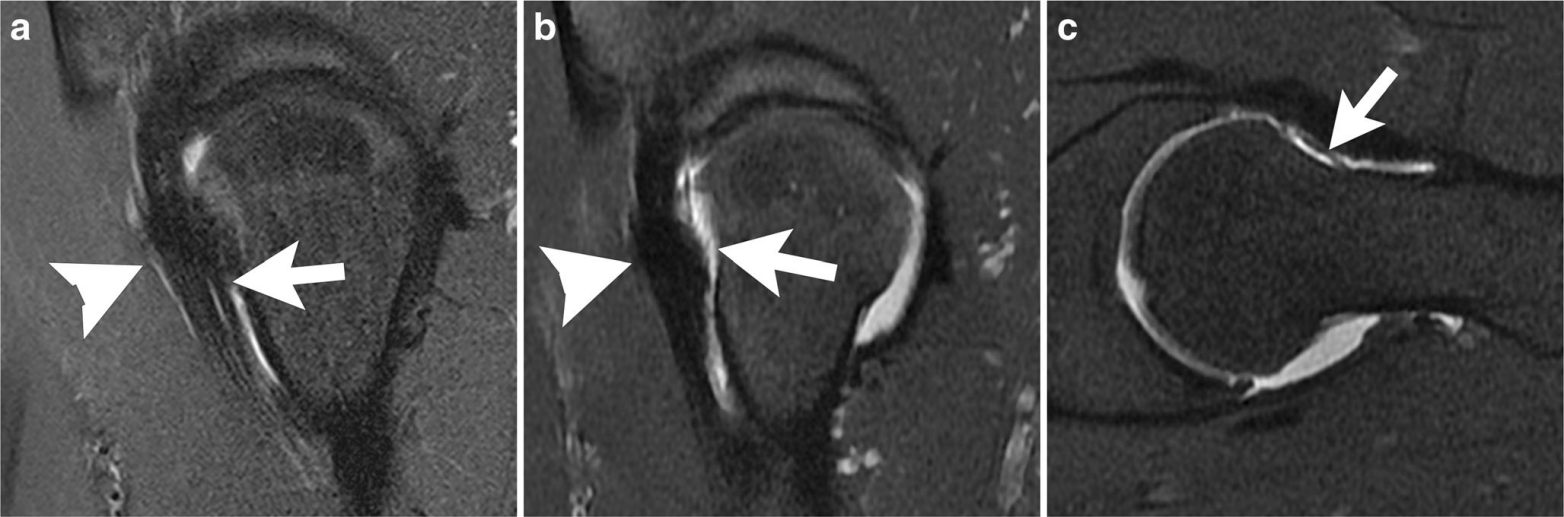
A 30-year-old man with a sensation of tightness and pain when fully extending his hip. The pain had been gradually increasing in the 1.5 years since his arthroscopy for FAI. a Sagittal PDFS MR at 3 T shows that the iliofemoral ligament is thickened (*arrowhead*) and there appears to be a fibrous strand (*arrow*) extending to the osteochondroplasty site. The surgeon felt that this might be a spurious finding related to the lack of joint distention; thus, MRI arthrography was subsequently performed. b Sagittal PDFS MR arthrogram at 1.5 T. Contrast material slightly separates the thickened iliofemoral ligament (*arrowhead*) from the anterior margin of the femoral neck, and facilitates recognition of fine strands between the capsule and the femoral head. c Axial oblique T1FS MR arthrogram at 1.5 T best shows the fibrous adhesions (*arrow*)
